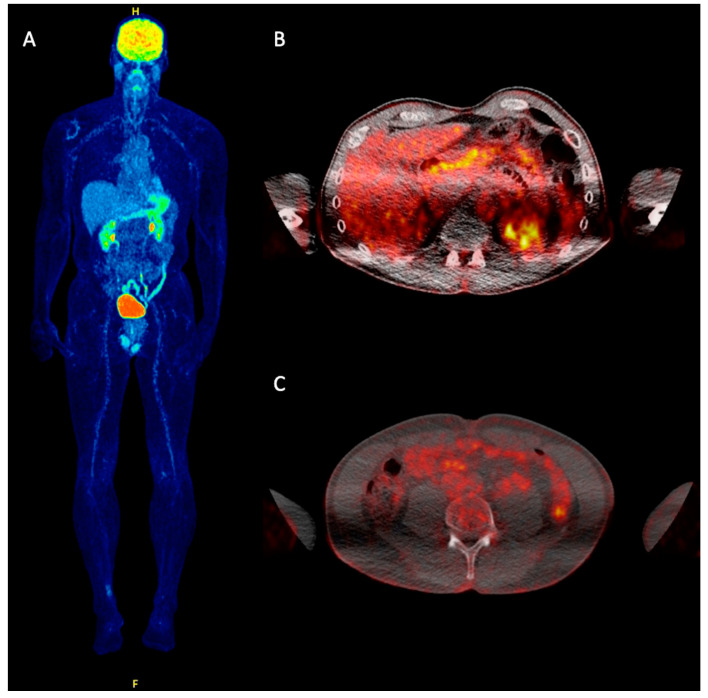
Post-treatment follow-up ^18^F-FDG PET/CT of the same patient as in Figure 1 after one year. Following anti-programmed death-ligand 1 (PD-L1) immunotherapy (avelumab), both whole-body MIP (A) and transaxial, fused ^18^F-FDG PET/CT images (B,C) demonstrated complete metabolic remission (CMR) of the previously observed metastases as response to treatment.
